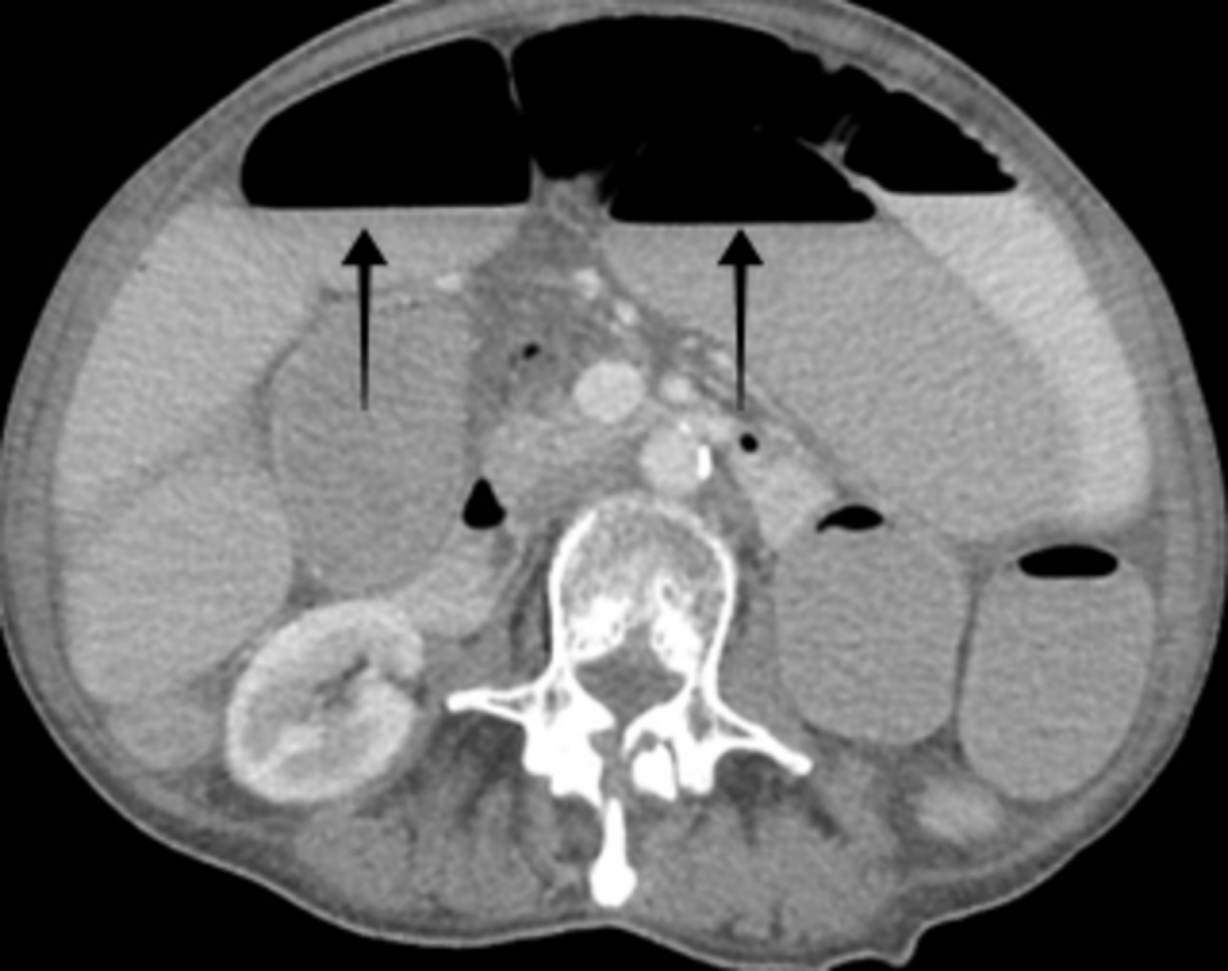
CT scan of the abdomen revealing high-grade small bowel obstruction with transition point noted centrally in the pelvis in the region of thickened small bowel loops. CT: computed tomography
